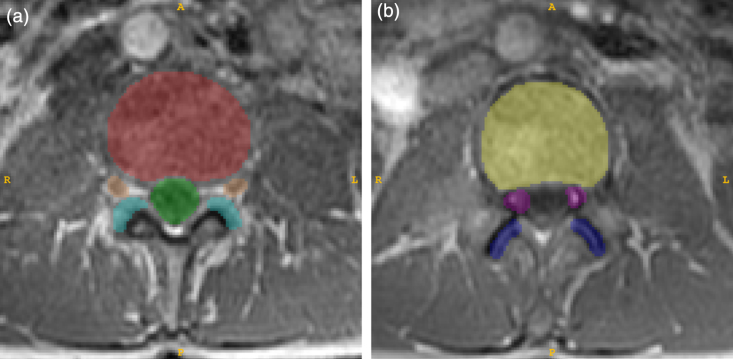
Example segmentations overlaid on the in-phase T1 weighted MRI. Images are cropped for viewing purposes. (a) Axial slice at the level of the intervertebral disc L3–L4, showing the disc (red), spinal canal (green), perifacet region (light blue), and nerve root (orange). (b) Axial slice at the level of vertebral body L3, showing the vertebral body (yellow), lateral recesses (purple), and facet joint (dark blue).
